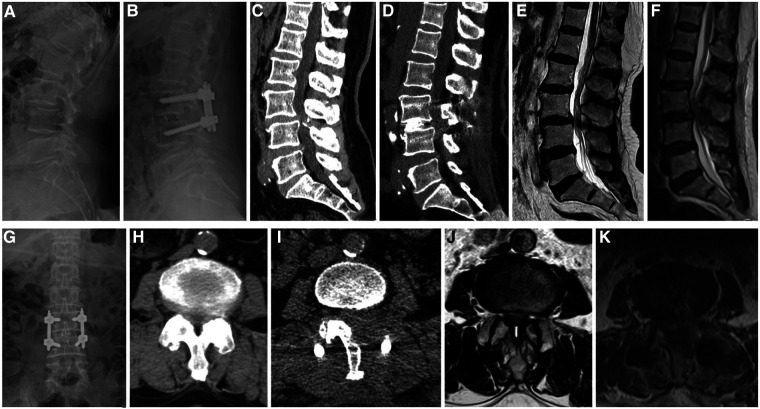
A 62-year-old female patient, whose complaints were low back pain since 3 years, lower limbs numbness, and intermittent claudication since 5 months. (A,C,E) Preoperative lateral radiographs, sagittal CT, and MRI showing instability of the L3 vertebral body, L3–4 spinal stenosis, and ossification of the posterior ligamentum flavum. (H,J) Preoperative axial CT and MRI showing significant spinal stenosis in L3–4. (B,G) Postoperative anteroposterior and lateral radiographs showing a good position of the instrumentation and the cage and improved segmental instability. (D) Postoperative sagittal CT showing that adequate bone was grafted. (F) Postoperative sagittal MRI showing that spinal stenosis was improved. (I) Postoperative axial CT showing unilateral laminectomy bilateral decompression. (K) Postoperative axial MRI showing sufficient decompression and a good position of the cage.
